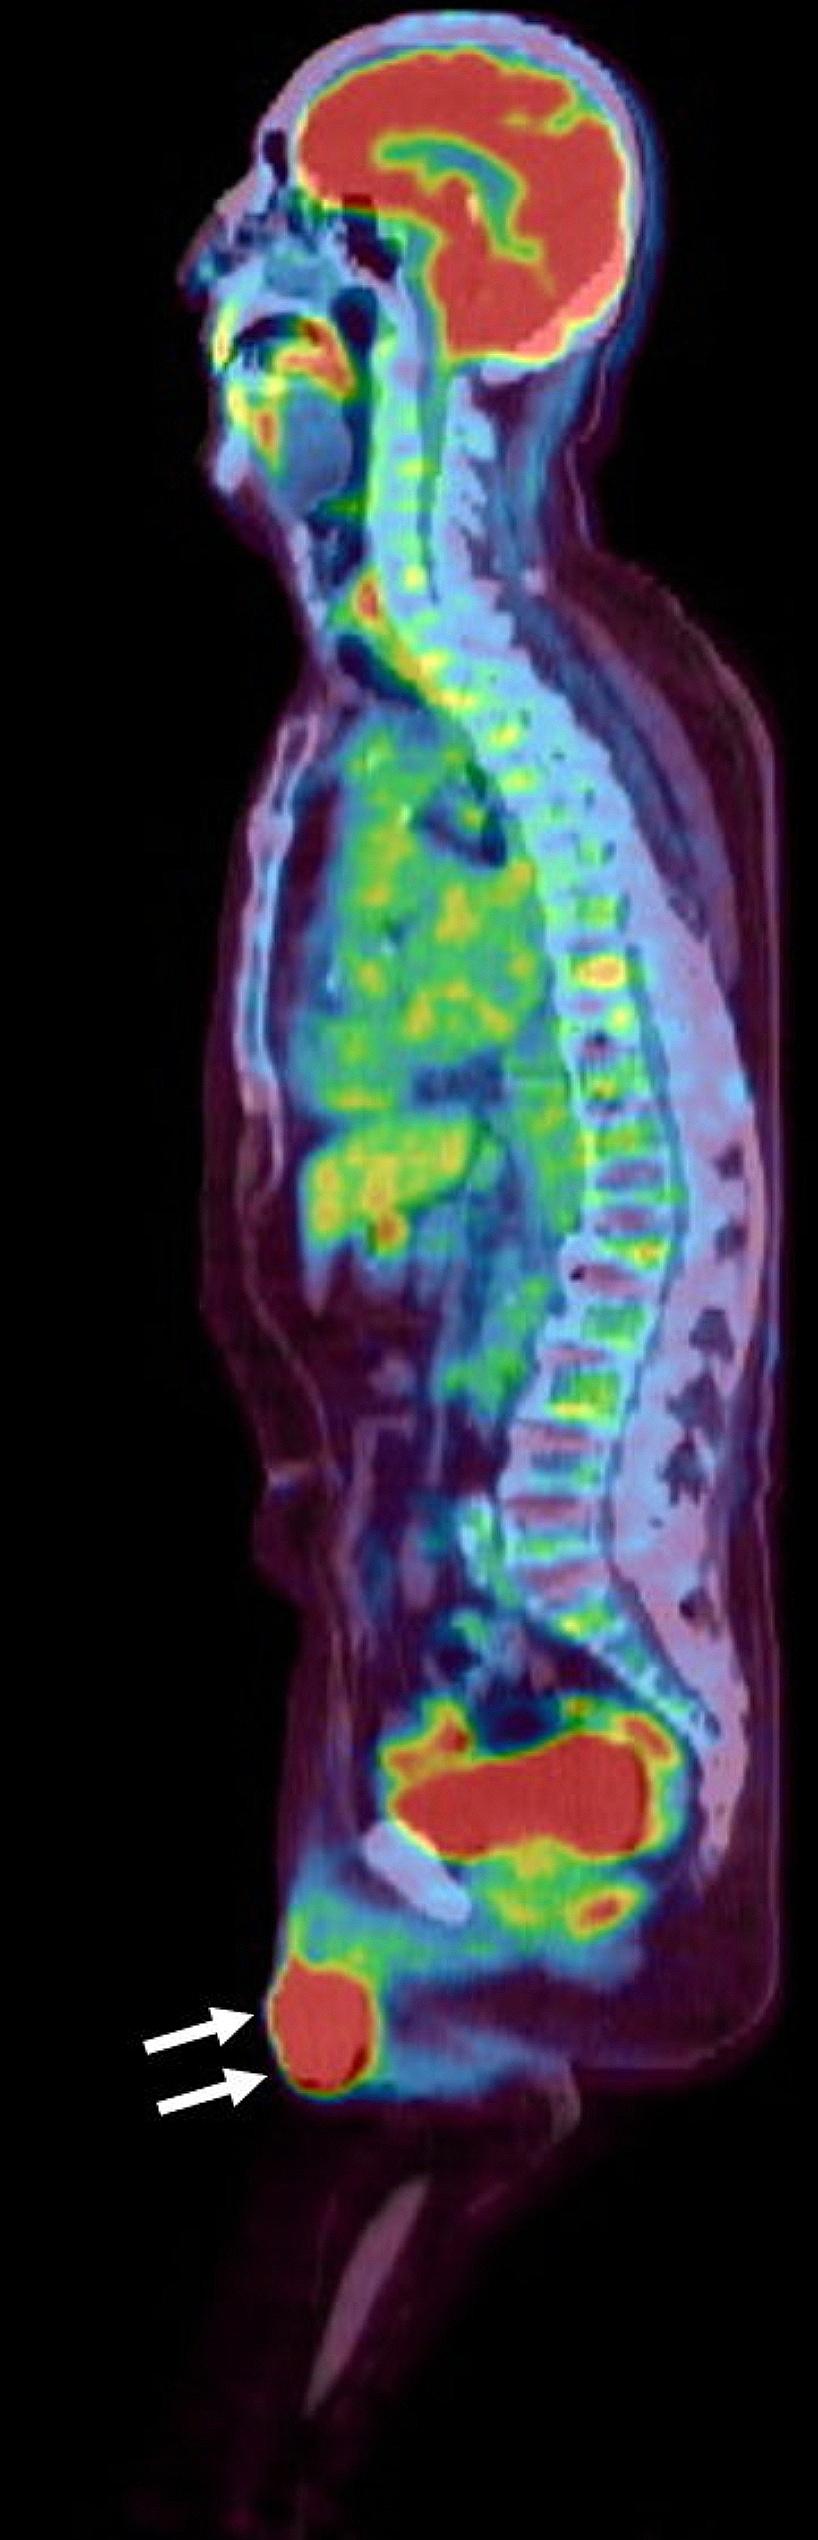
Sagittal fused fluorodeoxyglucose positron emission tomography/computed tomography image. High fluorodeoxyglucose uptake in the glans penile tumor was confirmed (white arrows; maximum standardized uptake value, 33.3)
